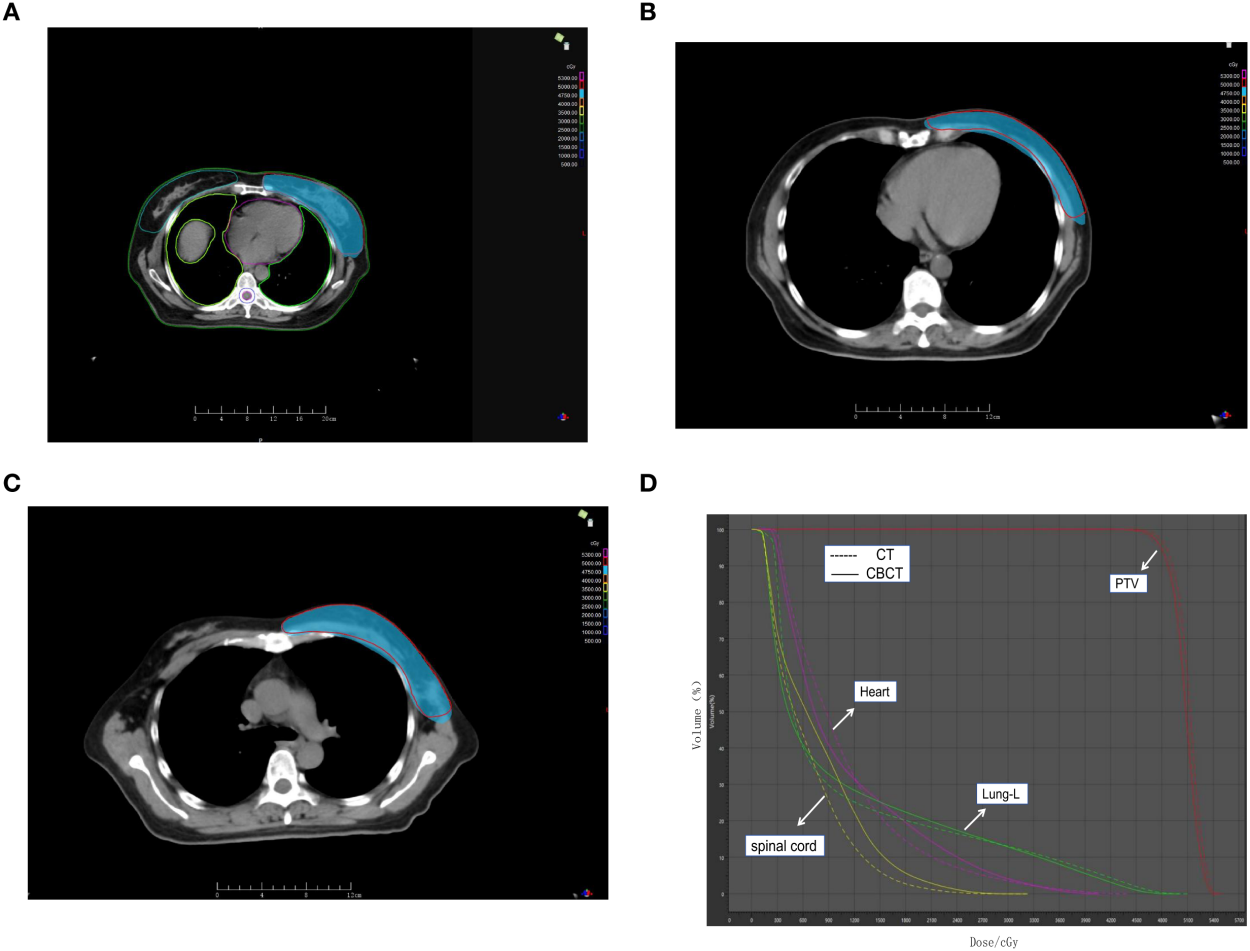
Representative dose distribution and DVH comparison between CT-based and CBCT-based plans. (A) Axial dose distribution of CT-based plan showing PTV coverage (B) Axial dose distribution of CBCT-based plan showing PTV coverage (C). Axial dose distribution showing delineated PTV and OARs (D) Comparison of Dose–Volume Histograms (DVH) for PTV and Organs at Risk (OARs) between CT-based and CBCT-based Plans.
